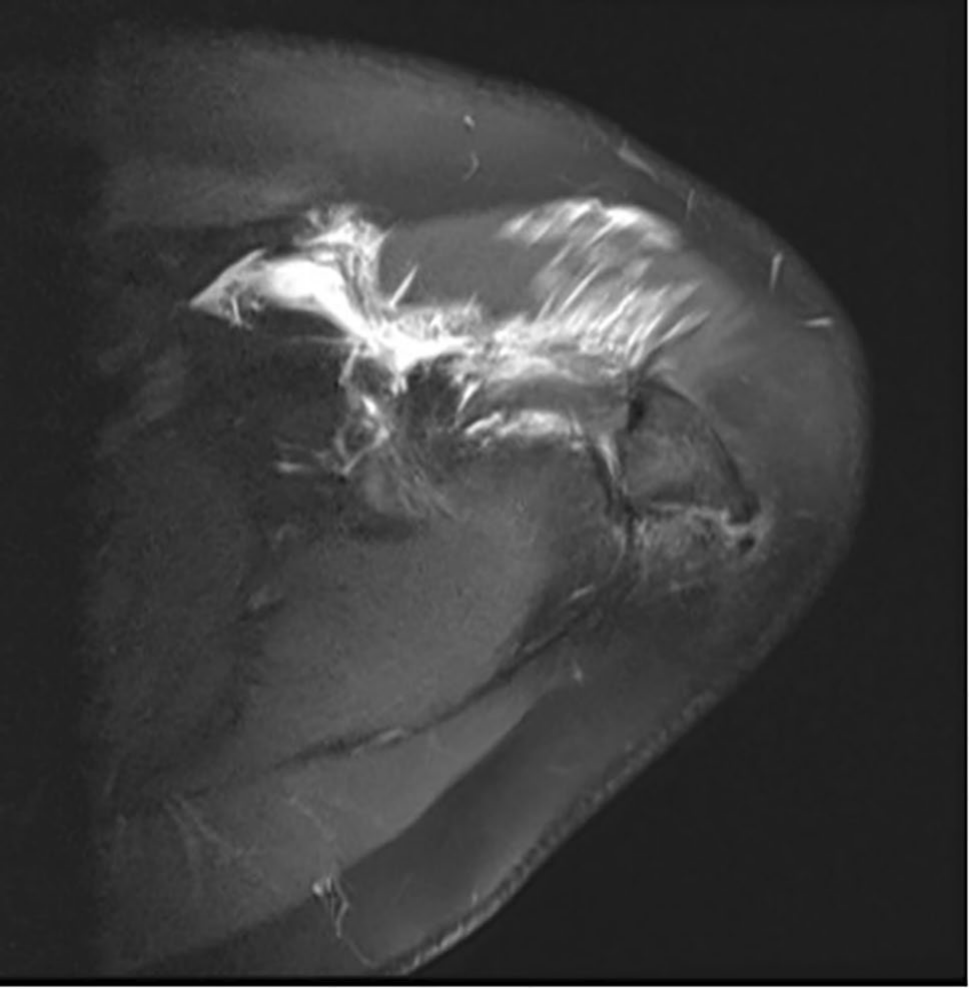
Magnetic resonance image depicting the os acromiale
